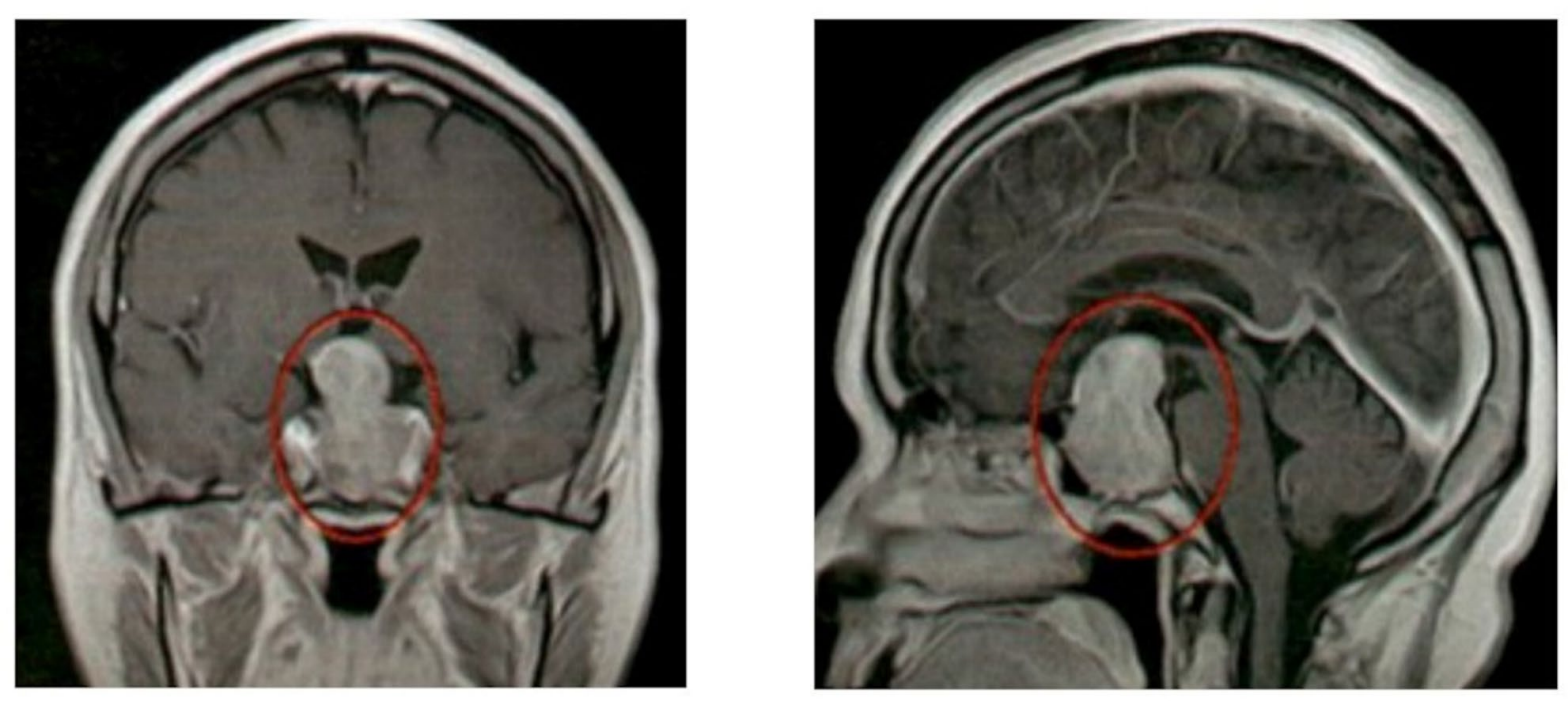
Magnetic resonance imaging before therapy. Magnetic resonance imaging of the brain showing a sellar and suprasellar mass requiring differential diagnosis of pituitary adenoma and clinically suspected to be pituitary metastasis from malignant melanoma. Red circles indicate the pituitary mass
